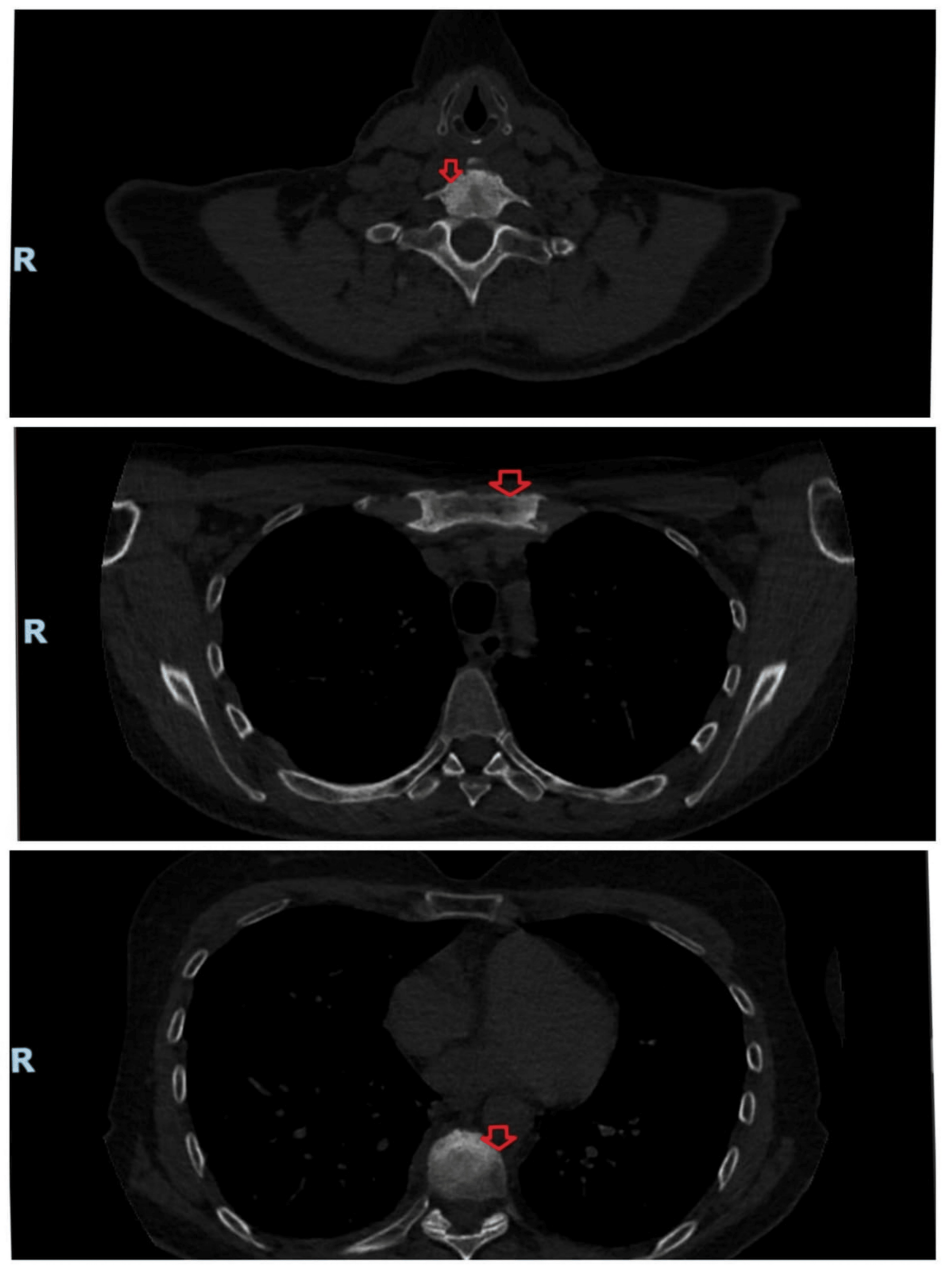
Follow-up axial CT Scan bone window shows subtle improvement in the appearance of the sclerosis within the sternum, lower cervical, and mid-thoracic spine.
